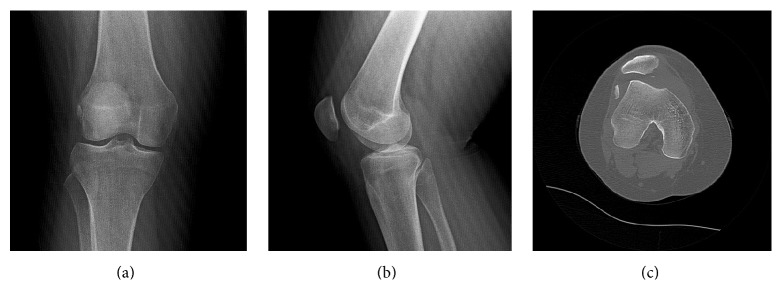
Preoperative anteroposterior (a) and lateral (b) X-rays and axial computed tomography (CT) (c) of the right knee show the free fragment and osteochondral defect.
